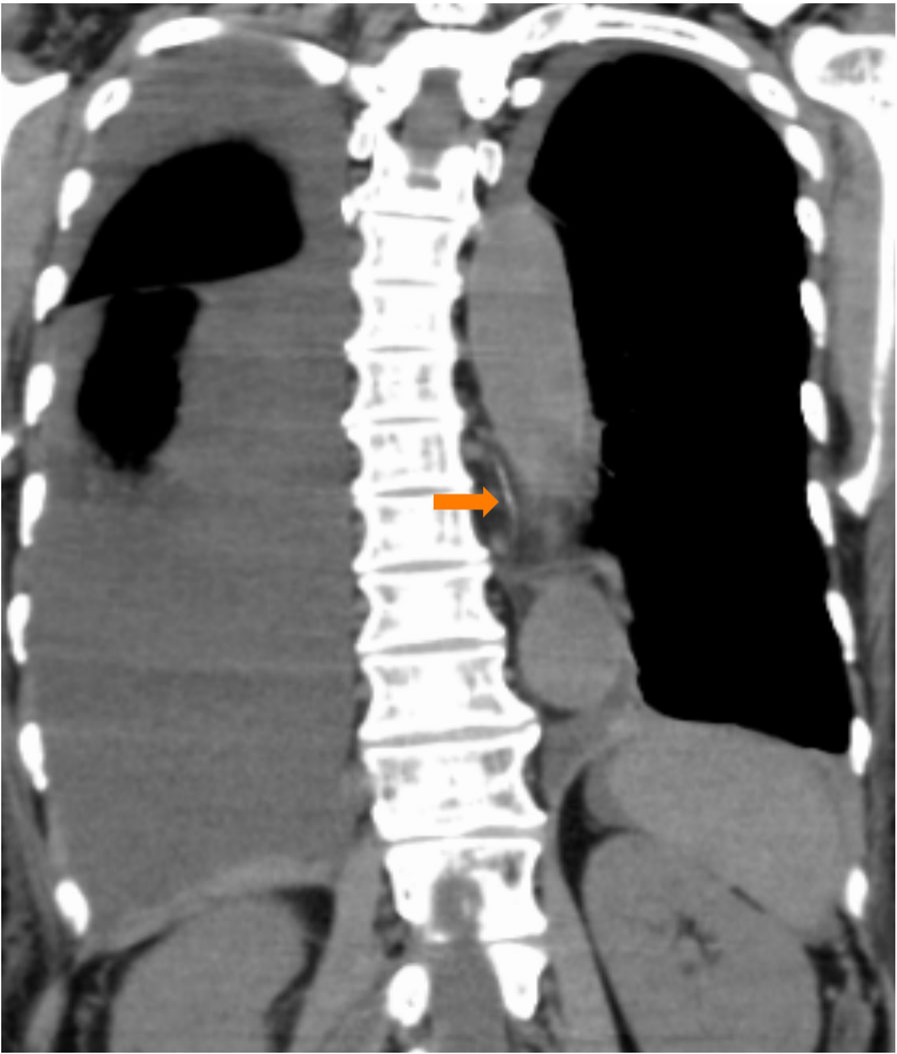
Post-procedure computed tomography after lymphangiography with Lipiodol (ethiodized oil) showing neither obstruction nor dilation of the thoracic duct. The arrow pointing to the thoracic duct
